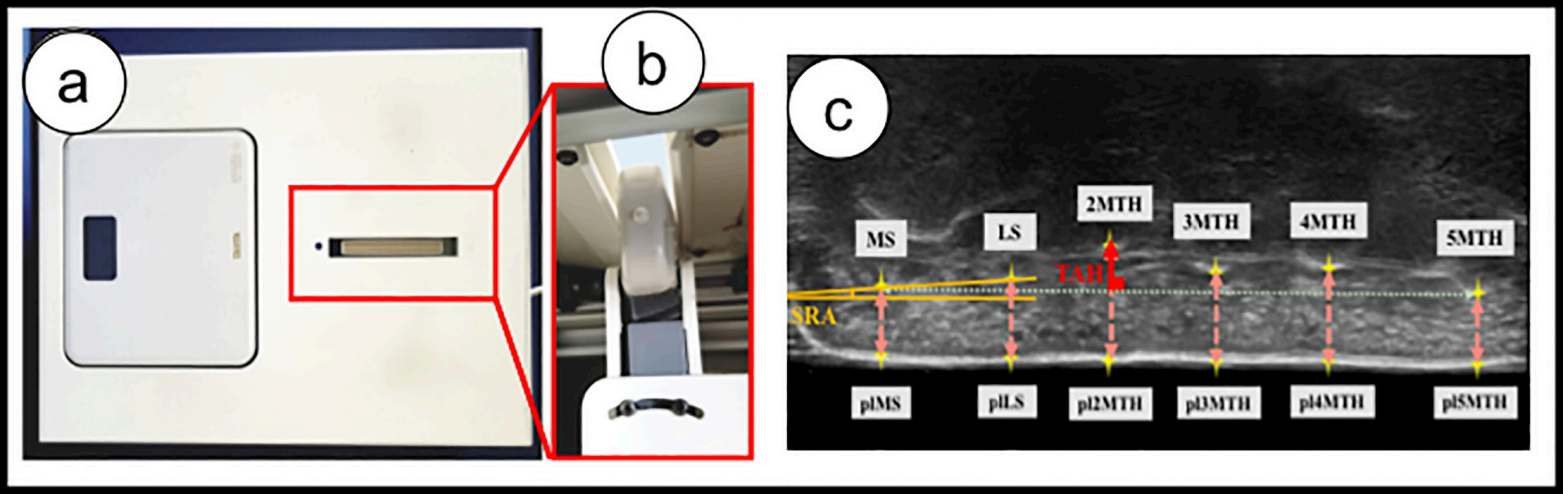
Weight-bearing plantar ultrasound imaging device and ultrasound images. (a) WPUID constructed with an internal probe allowing coronal views of the transverse arch; (b) ultrasound probe inserted upside-down and an upper opening for a gel pad to be placed on the probe; (c) shows the ultrasound image: the lowest point of the MS, LS, 2MTH, 3MTH, 4MTH and 5MTH as well as their plantar projections were marked by yellow stars. TAH (red line) is the distance from 2MTH perpendicular to the line passing through MS and 5MTH. Metatarsal heads’ height (orange dotted lines) is the distance between the lowest point of the bone and its plantar surface marker. SRA (yellow angle) is the angle between the line passing through MS and LS and the line passing through MS and 5MTH. US: ultrasound; WPUID: Weightbearing Plantar Ultrasound Imaging Device; MTH: metatarsal head; TAH: transverse arch height; SRA: sesamoid rotation angle; MS: medial sesamoid; LS lateral sesamoid; 2MTH: second metatarsal head; 3MTH: third metatarsal head; 4MTH: forth metatarsal head; 5MTH: fifth metatarsal head; pl: plantar surface.
